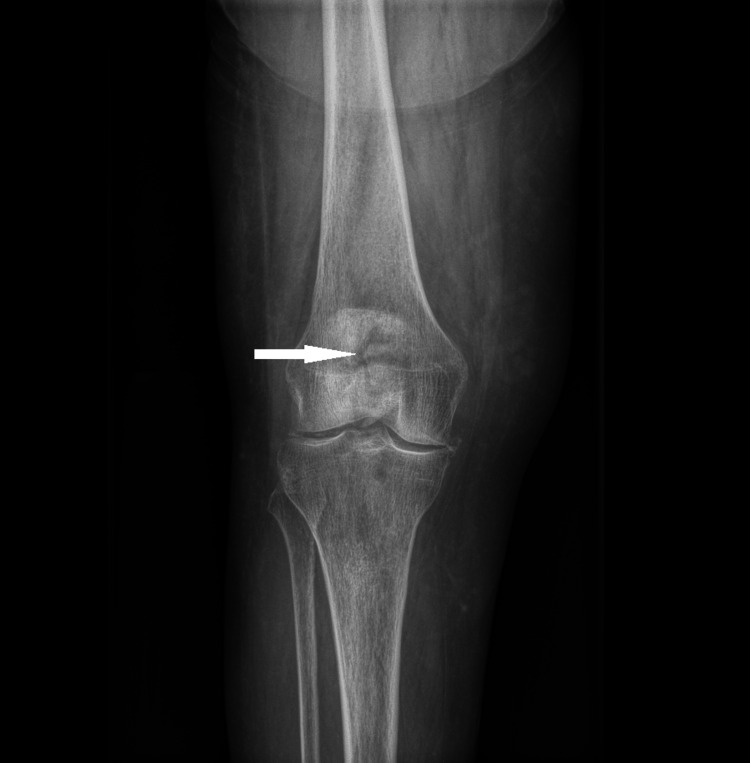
Coronal view X-ray of the patient's right knee demonstrating a comminuted fracture of the patella demonstrated by the hypodense area on the anterior aspect of the patella.
